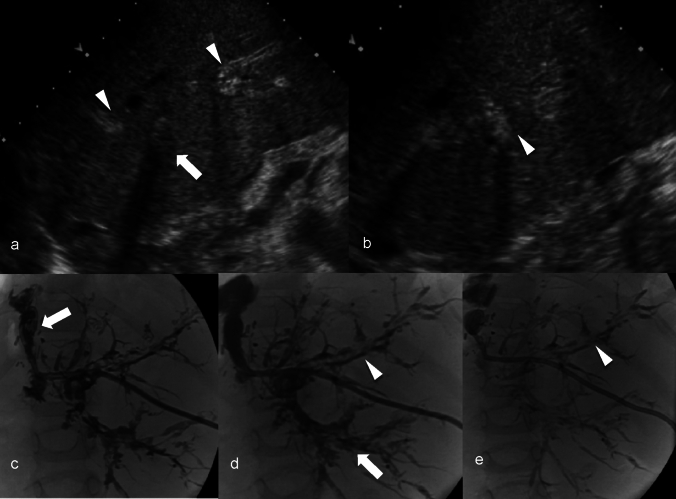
Ultrasound images demonstrate multiple echogenic foci scattered in the liver within the portal triads (arrowhead, a–b) related to inflammation around the biliary ducts with a few bile ducts demonstrating posterior acoustic shadowing due to ductal stones (arrow, a–b) in a patient with history of recurrent cholangitis, fevers, and massively elevated alkaline phosphatase. Endoscopic retrograde cholangiopancreatography images demonstrate diffusely irregular and dilated ducts (arrow, c–d) with filling defects (arrowhead, d–e)
